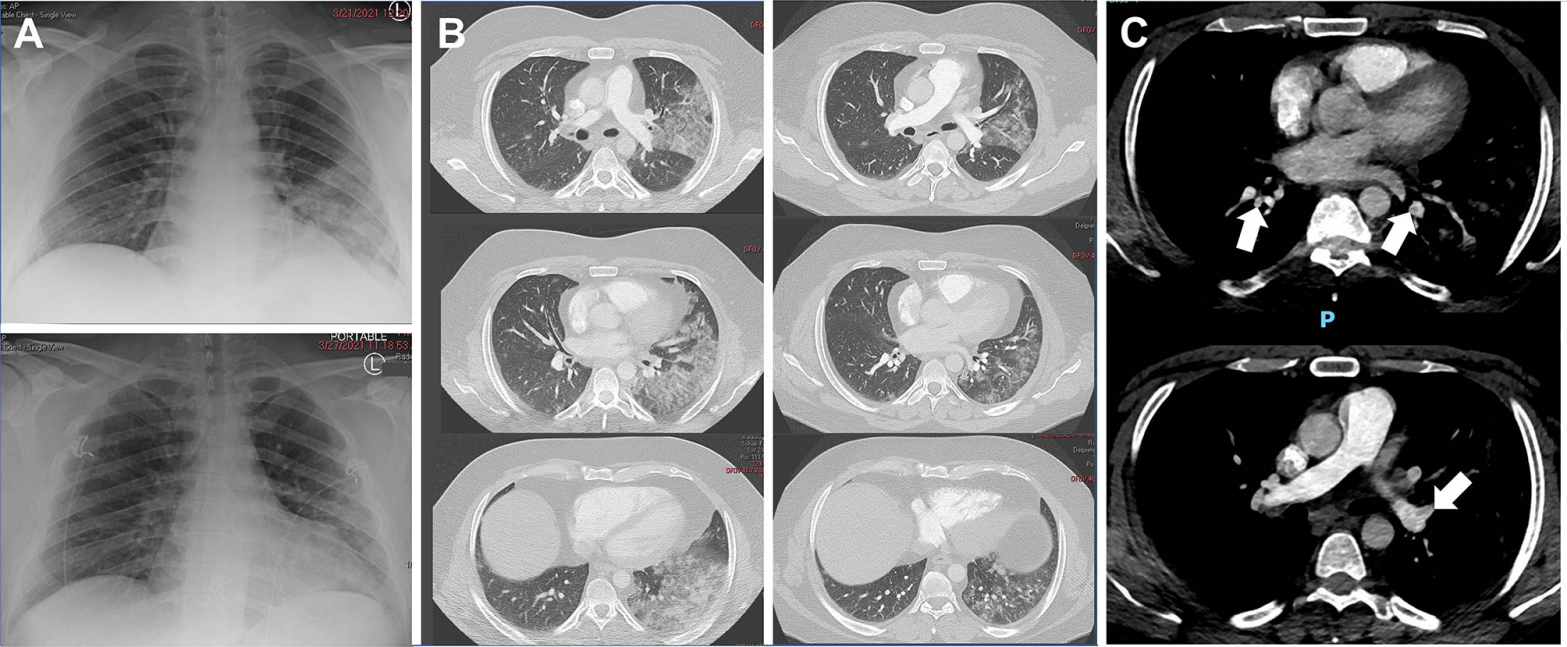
Temporal evolution of radiographic abnormalities in Patient 1 during the course of COVID-19. (A) Chest X-ray demonstrating left lower lung airspace disease on admission at the NIH Clinical Center (upper panel) and radiographic improvement noted 7 days later (lower panel). (B) Chest CT showing left>right pulmonary infiltrates on admission at the NIH Clinical Center (Left panels) and radiographic improvement noted 7 days later (right panels). (C) Chest CT angiography on admission at the NIH Clinical Center showing small pulmonary emboli (white arrows).
